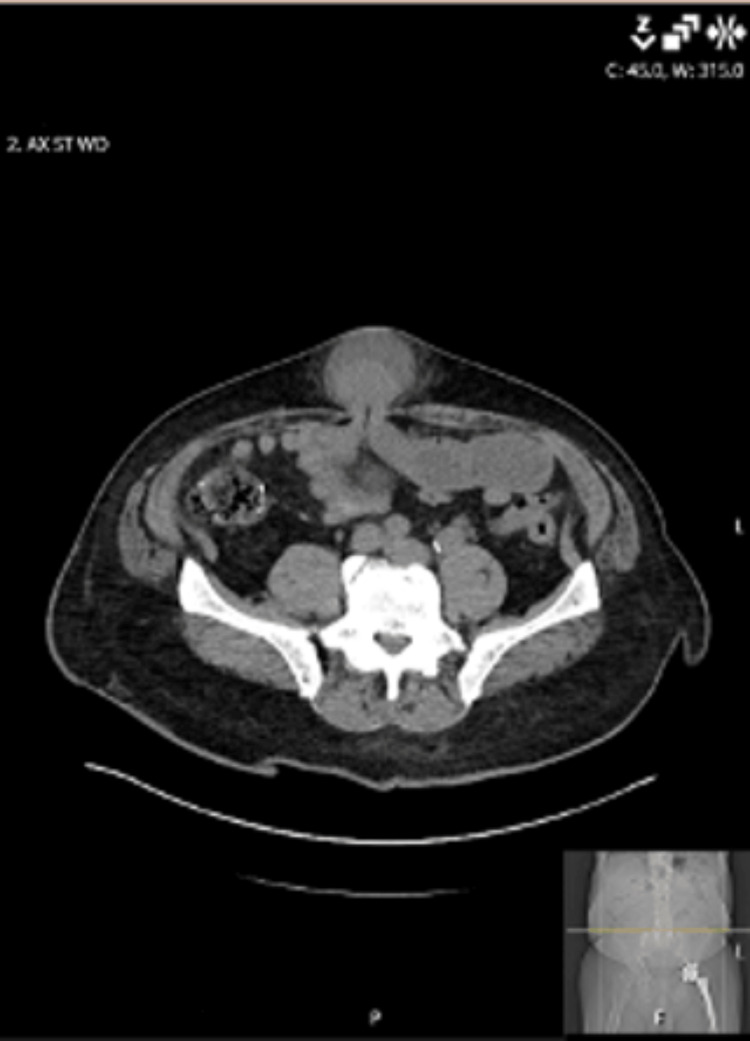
Axial computed tomography image demonstrating an umbilical hernia containing the small bowel and omentum
